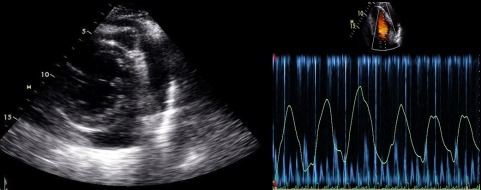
Parasternal short axis view (left panel) showing a circumferential pericardial effusion surrounding the left ventricle. Pulse wave Doppler (right panel) with respirometry of mitral inflow demonstrating the pronounced respiratory variation in filling.
